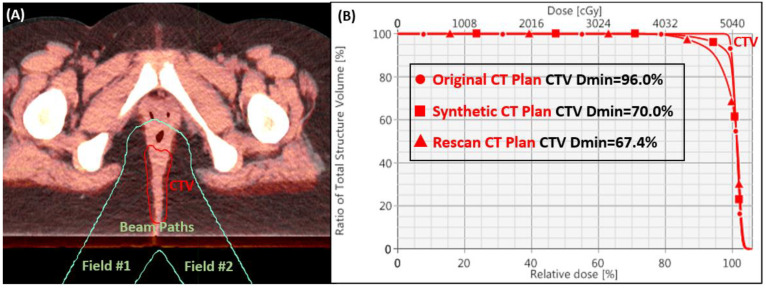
The tissue discrepancy of 12 mm was shown in the overlaid axial plane of the original and rescanned CTs in (A). The impact of plan quality was demonstrated for CTV Dmin in the DVH curves for original, rescanned, and synthetic CT plans in (B) for patient #1.
